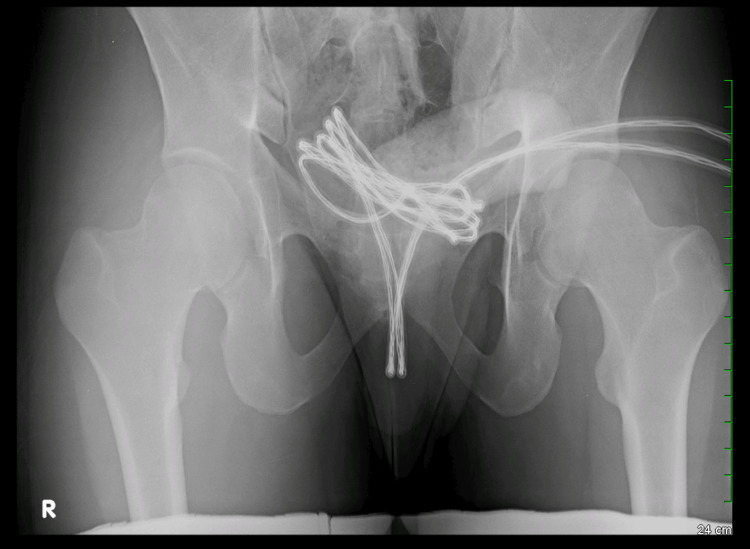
KUB radiograph depicting position of foreign body in urethra and bladder. KUB: kidney, ureter, and bladder
